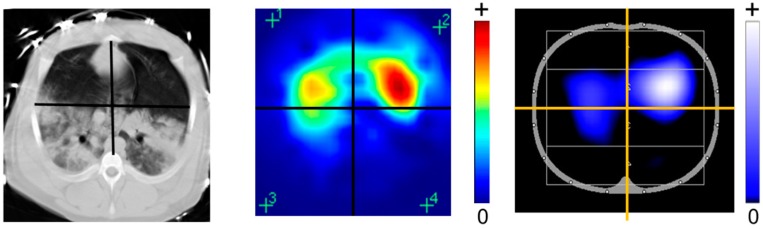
Different available color codings of EIT images in comparison to the CT scan. The rainbow-color scheme uses red for the highest relative impedance (e.g., during inspiration), green for a medium relative impedance, and blue for the lowest relative impedance (e.g., during expiration). A newer color scales use instead black for no impedance change), blue for an intermediate impedance change, and white for the strongest impedance change.
